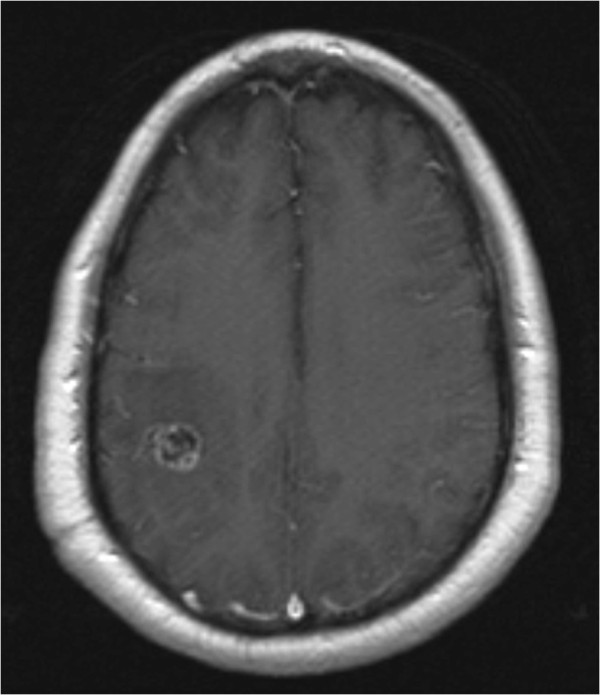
Contrast-enhanced axial T1-weighted image revealing a heterogeneous contrast-enhancing lesion in the subcortical white matter of the right parietal lobe and no apparent dural attachment.
